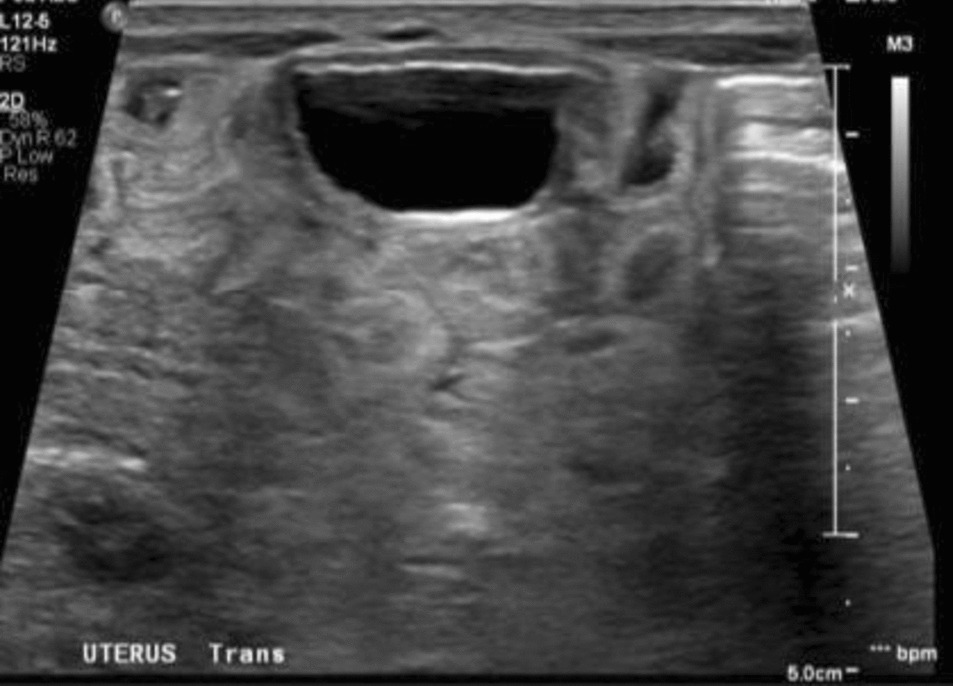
Abdominal ultrasound showing bicornuate uterus
